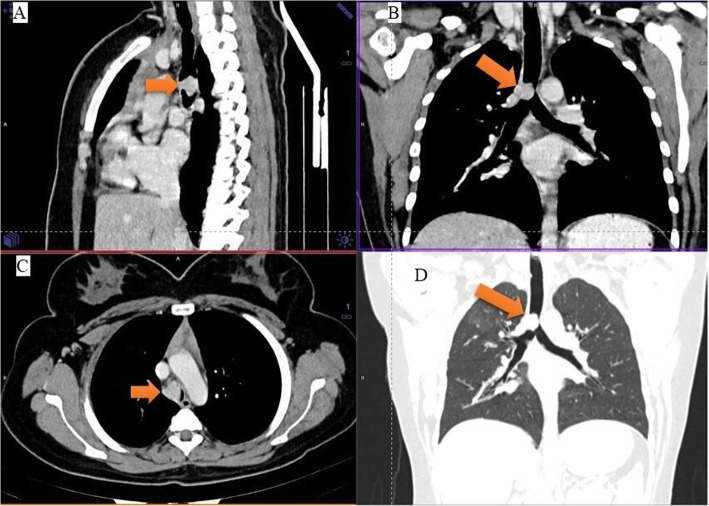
Sagittal (a), coronal (b), axial (c), and lung window (d) views of CT scans of thorax show a well-defined polypoidal soft tissue density lesion (solid yellow arrows) on the right posterolateral side of trachea at T4 vertebral level with gross luminal compromise, just proximal to carina with no extra luminal extension, laryngotracheal deviation, and lung collapse
